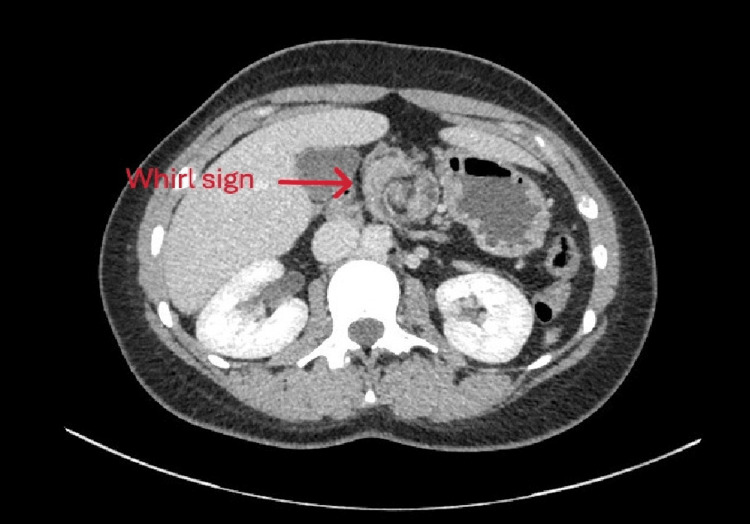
Abdominal CT scan showing a whirl sign, suggesting a volvulus
